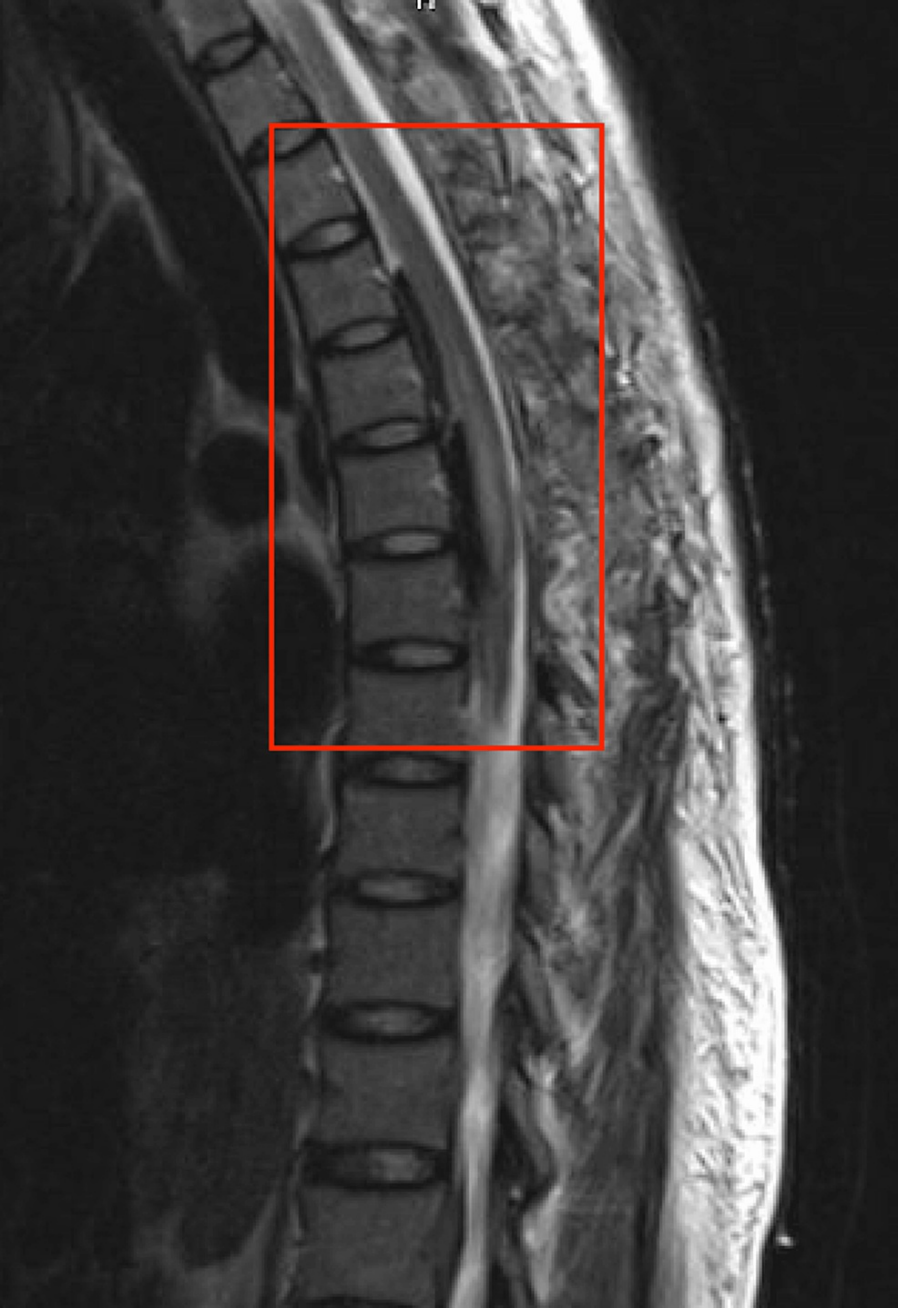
T1-weighted MRI of the thoracic spine portrays the epidural hematoma that ranged from T2 to T8.
